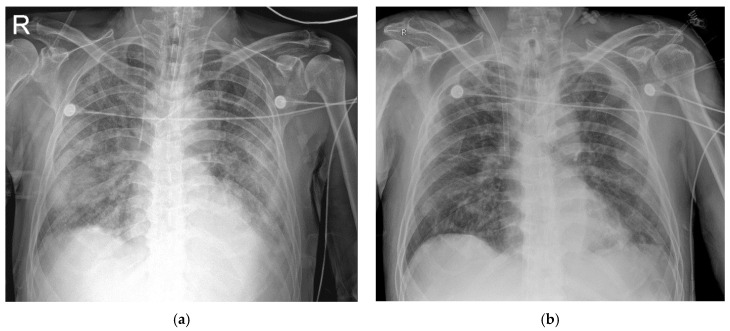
Chest radiography of the patient 1. (a) on the day of ECMO application (b) on the day of ECMO discontinuation.
